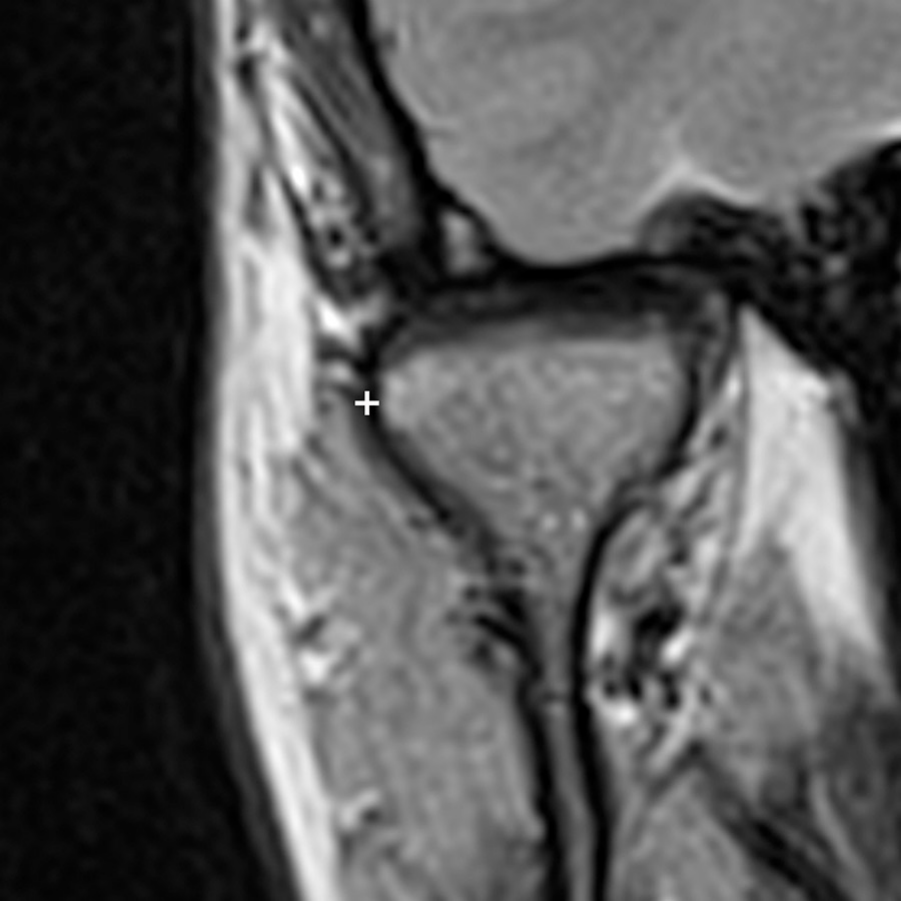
MRI of a right condyle in coronal view. The reference point on the condylar cortical (+) used to measure the LPAS is the most lateral cortical point at the condylar level. LPAS, lateral periarticular space.
